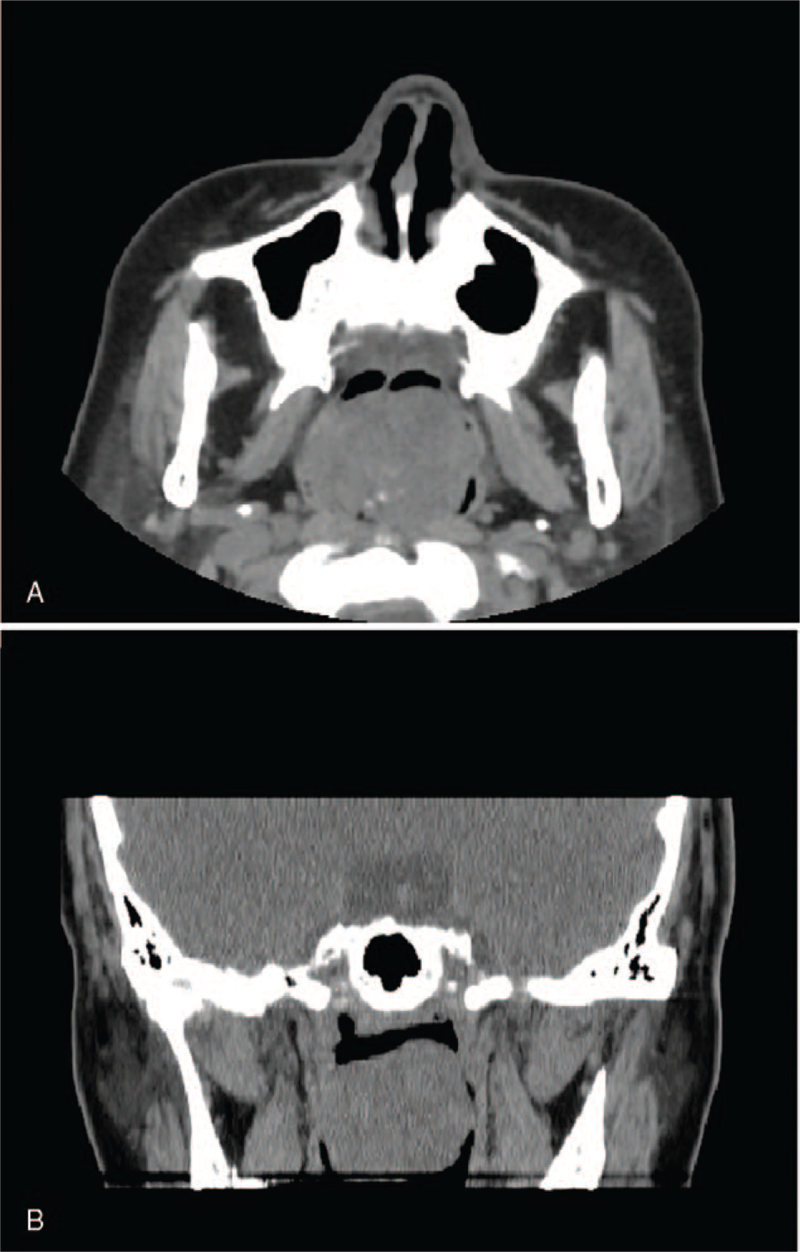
(A) Horizontal view image of head/neck CT scans shows a protruding mass in the nasopharynx (arrow). (B) A coronal view image of head/neck CT scans shows a protruding mass in the nasopharynx (arrow). CT = computed tomography.
